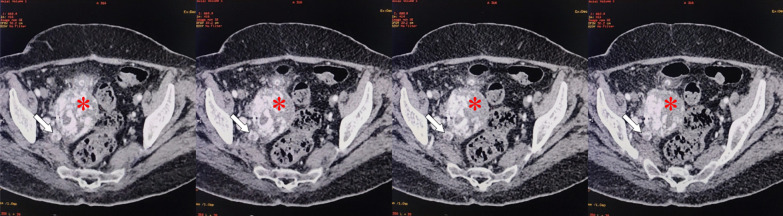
Abdominal CT axial scans showing the urinary bladder tumor (red star) extending into the right internal iliac vein (white arrow)
